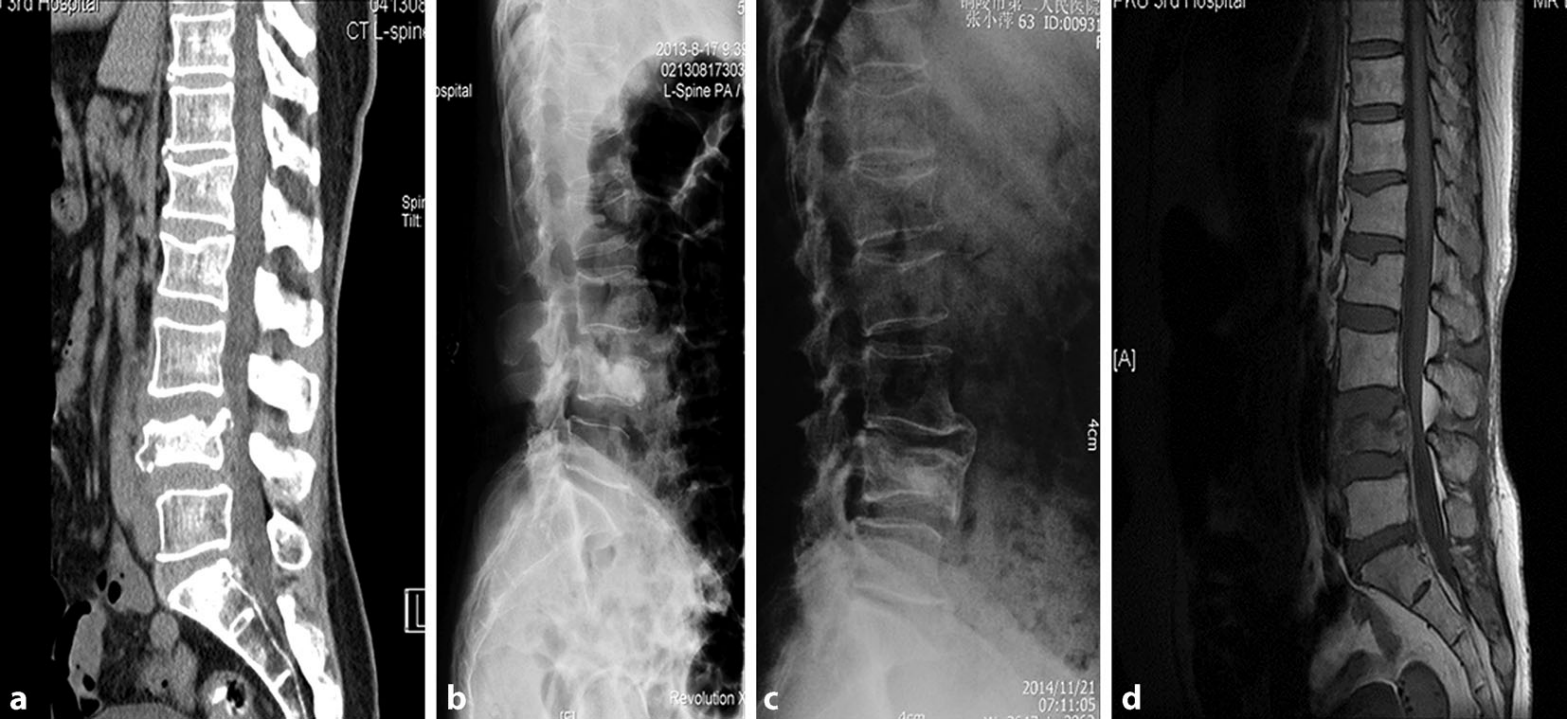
Radiograph (magnetic resonance imaging [*MRI*], computed tomography [*CT*], and X‑ray) images of typical case treated with temporary unilateral pedicle screw reduction with percutaneous kyphoplasty: a perioperative CT, b postoperative X‑ray, c postoperative 15-month follow-up X‑ray, d perioperative MRI
